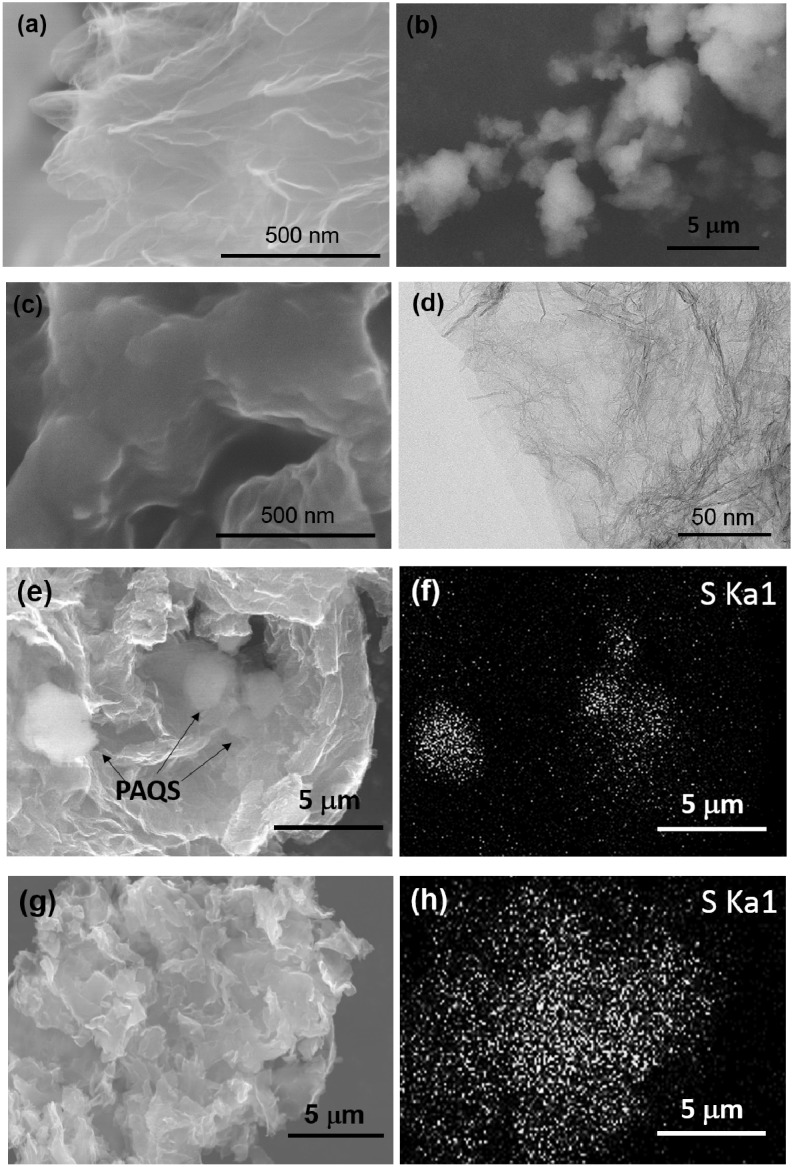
SEM images of (a) GSs; (b) PAQS; and (c) PAQS/GSs; (d) TEM image of PAQS/GSs. SEM images and energy-dispersive X-ray (EDX) sulfur elemental mapping images of (e,f) PAQS/GS(m) and (g,h) PAQS/GS.
